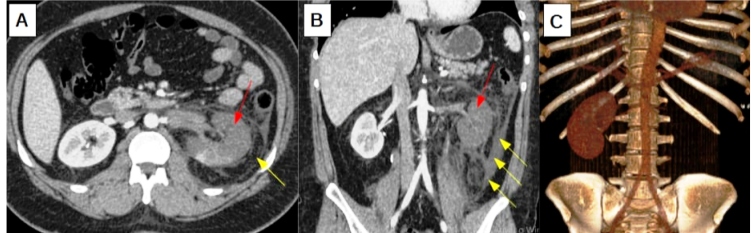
(A-C): Enhanced abdominal computed tomography. Left renal arterial thrombosis, with a marked reduction in renal perfusion (red arrows), with only a minimal uptake foci in the lower and middle third of this kidney. Important densification of the perirenal adipose planes with thickening of the anterior and posterior pararenal fascia as well as lateroconal fascia (yellow arrows).
